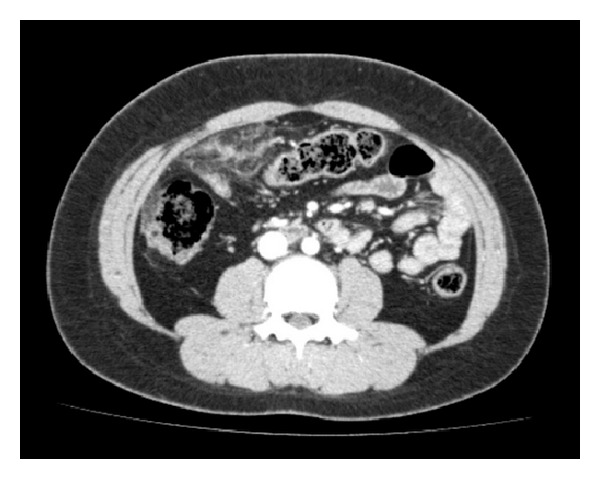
Enhanced CT reveals a 5 × 8 cm ill-defined heterogeneous fatty mass with hyperattenuating streaks just beneath the abdominal wall anterior to the transverse colon.
